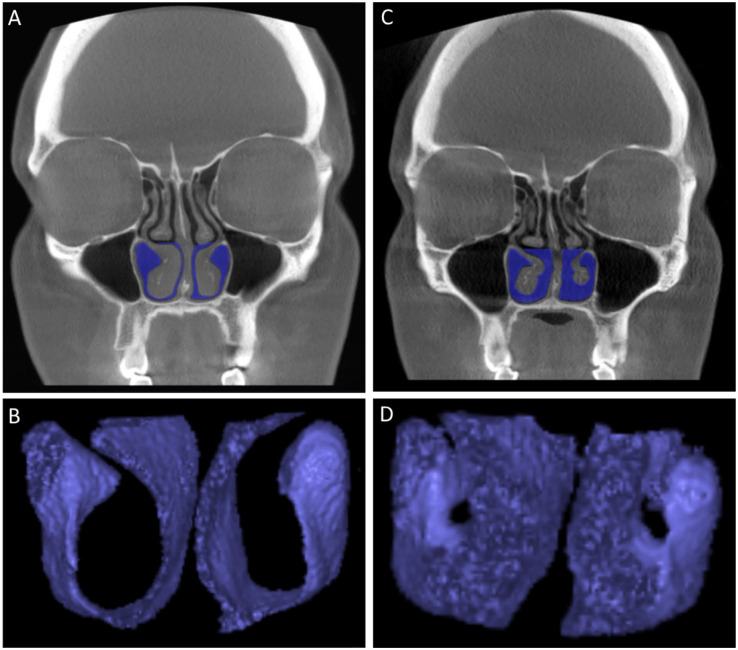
An example of the preoperative patient CBCT scans (A) with marked air spaces
